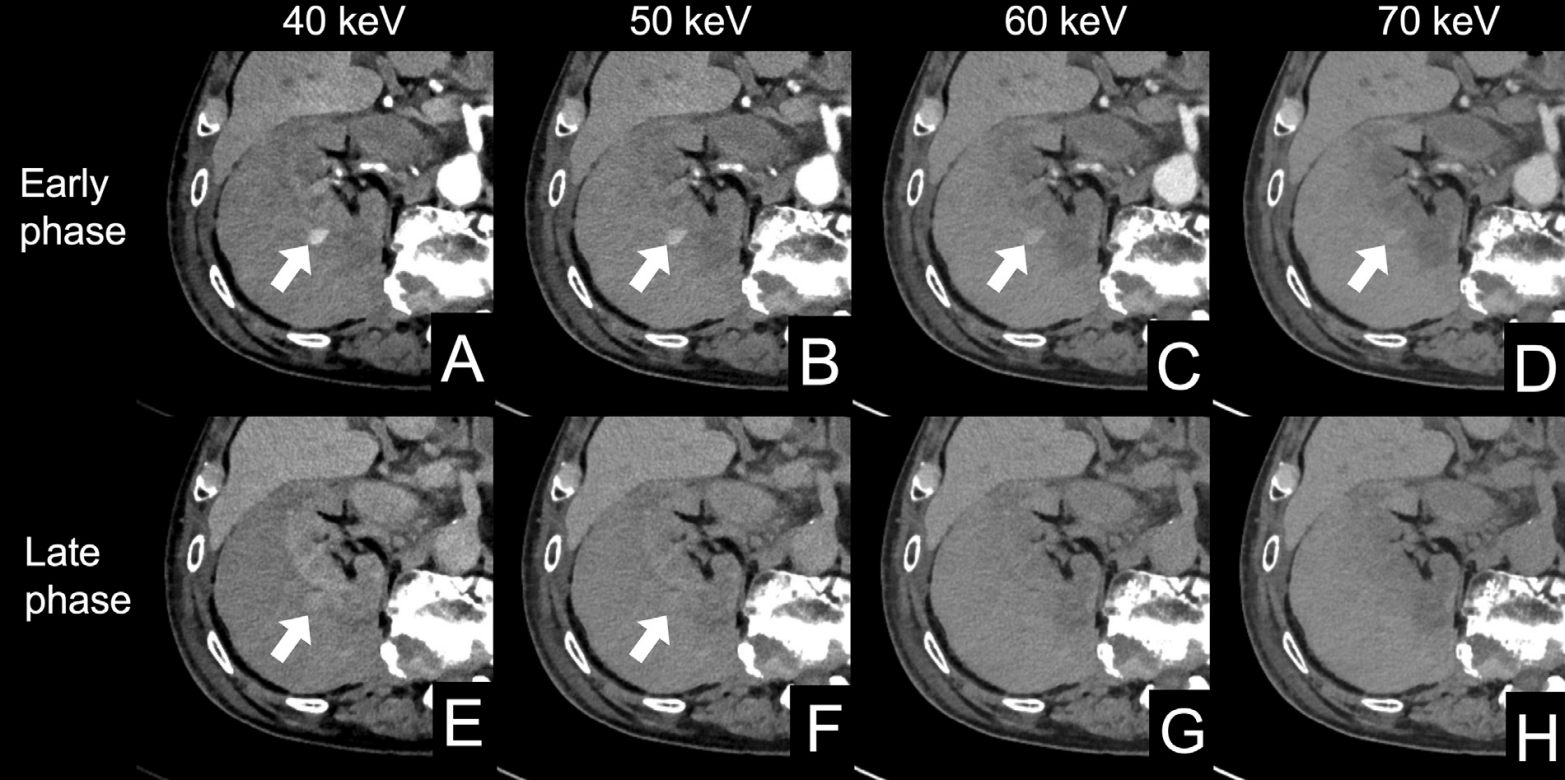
Comparison of VMI of the renal pseudoaneurysm at different keVs in early (A-D) and late (E-H) phases. The early phase at 40-70 keV (A-D) and late phase at 40, 50 keV (E, F) of contrast-enhanced images show an enhancing area corresponding to the nodular low-density area on non-enhanced CT (arrows). The enhancement of the renal pseudoaneurysm is delineated more at 40 keV than at higher keVs. On late phase contrast-enhanced images, the renal pseudoaneurysm is undetectable at 60 and 70 keV (G, H).
